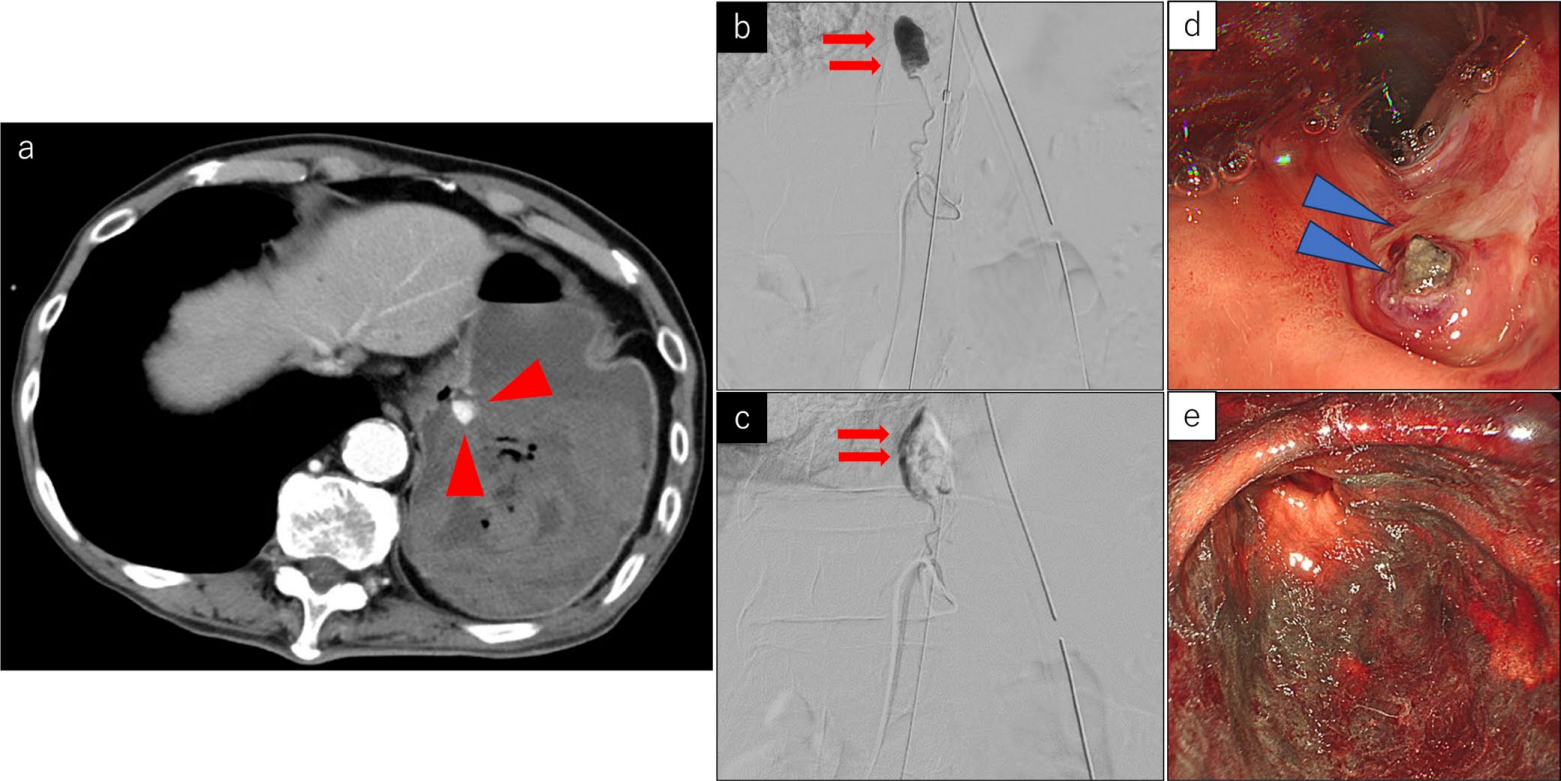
Contrast‐enhanced computed tomography (CT) scan on arrival, transcatheter arterial embolization (TAE), and upper endoscopy. (a) CT scan shows massive bleeding into the stomach. Red arrowheads show a large amount of extravascular leakage into the stomach. (b) Before TAE to the left gastric artery branch (red arrows). (c) After TAE to the left gastric artery branch (red arrows). d: Gastric ulcer with exposed blood vessels (blue arrowheads). e: The stomach showing ischemic changes.
